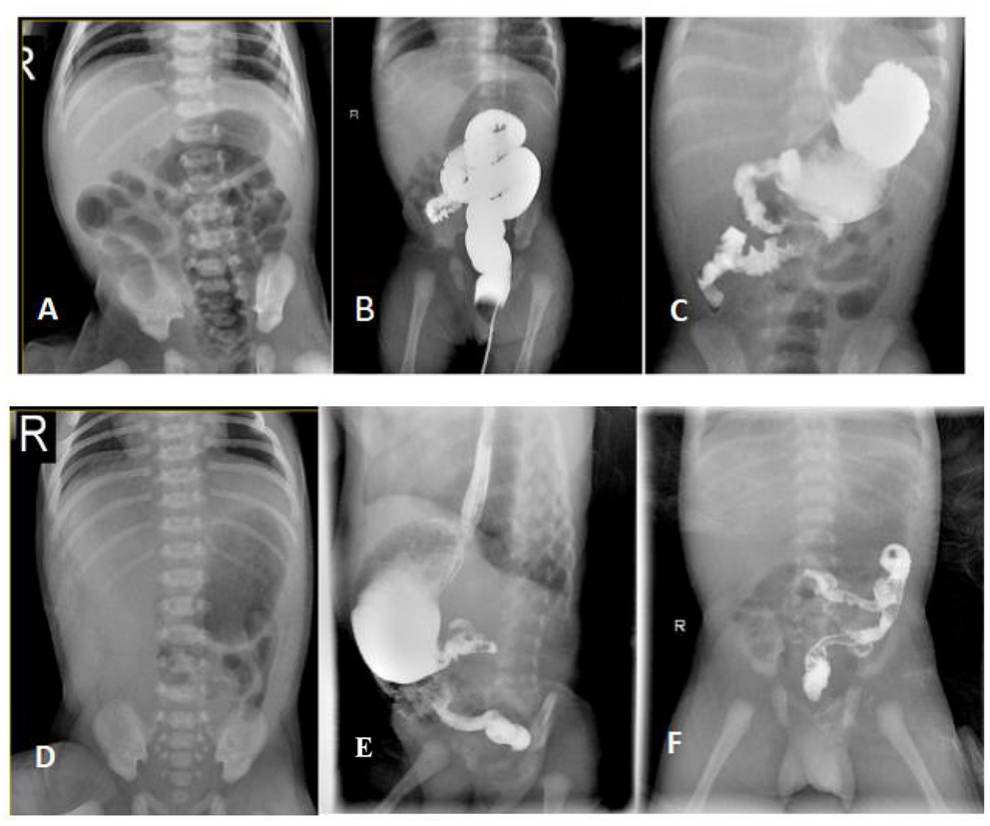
Radiological findings of the patients with CSBS. [The proband] (A) X-ray abdomen showing the gas-filled stomach and the intestinal loops; (B) Lower gastrointestinal radiography showing the distribution of the colon predominantly in the center and on the left side of the abdomen; (C) UGI showing the upper part of the jejunum located on the right side of the abdomen in a spiral pattern. [The younger brother] (D) X-ray abdomen showing minimal gas in the stomach with no obvious gas shadows in the intestine. (E,F) UGI showing the ascending part of the duodenum and the upper part of the jejunum in a spiral pattern.
